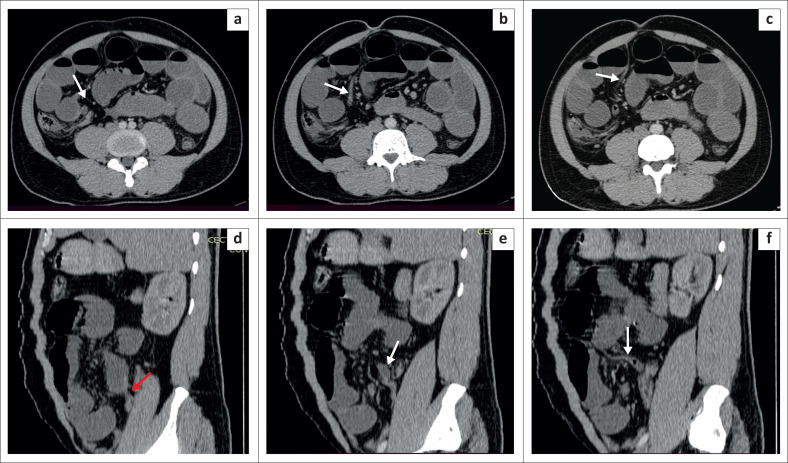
Contrast enhanced computed tomography in the axial (a, b, c) and coronal (d, e and f) planes show dilated small bowel loops with air-fluid levels. An inflamed Meckel’s diverticulum (white arrows) was seen arising at the level of the transition point (red arrow). An enhancing linear band was seen arising from the Meckel’s diverticulum, extending towards umbilicus (white arrow in f).
